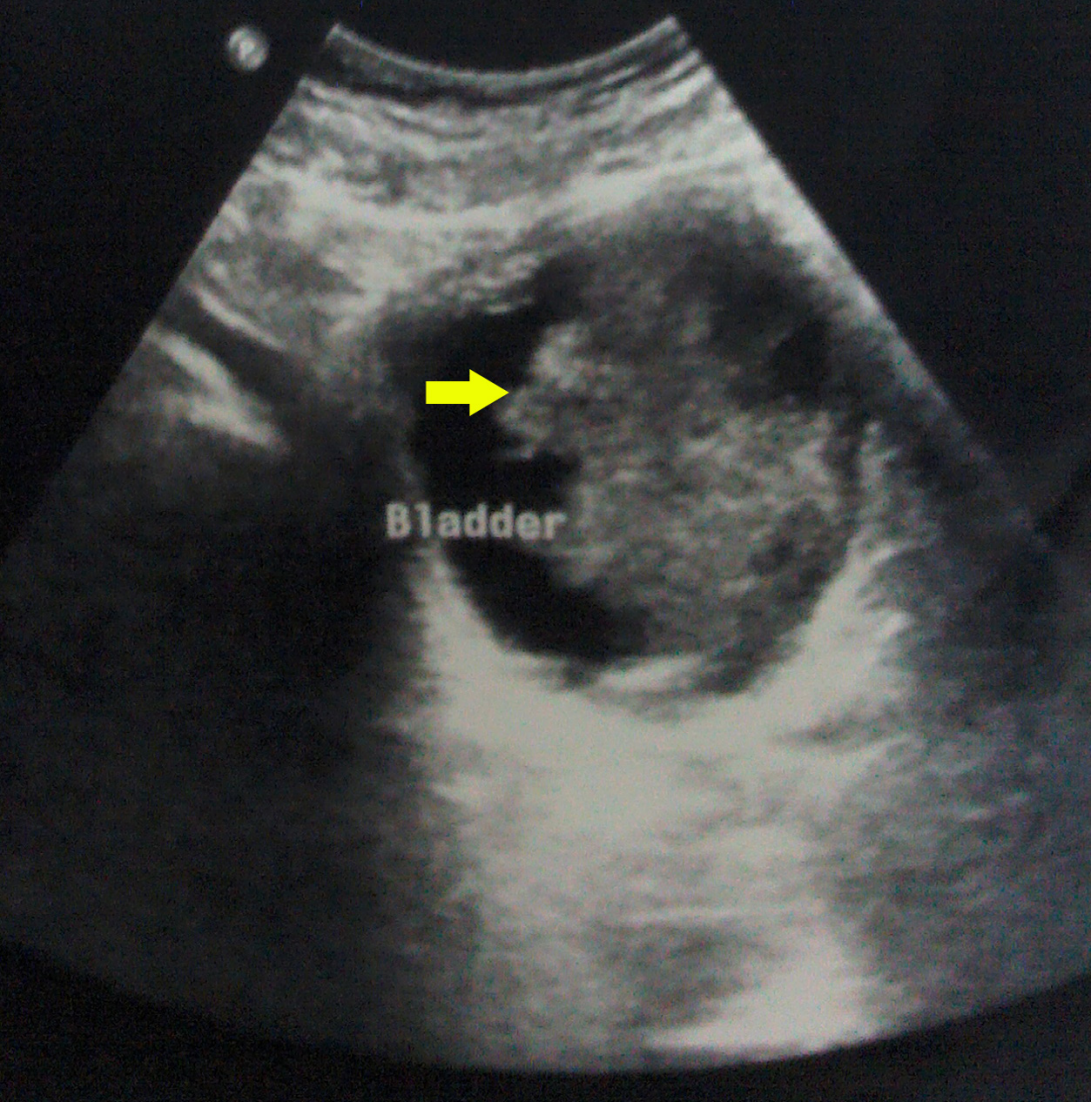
Ultrasonography showing a large echogenic irregular mass lesion with internal vascularity in the left lateral wall of the bladder projecting into the lumen.
